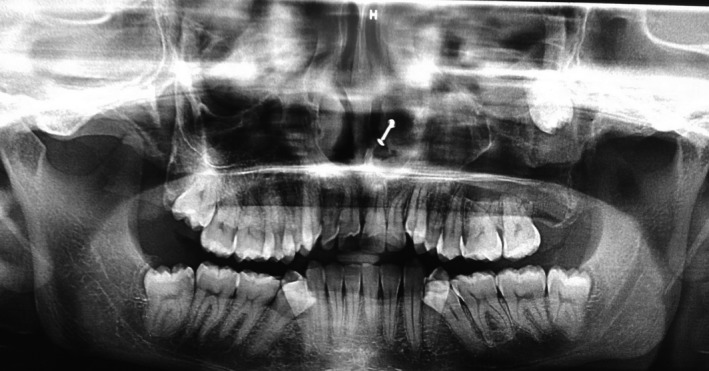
Orthopantomogram showing the presence of an ectopic tooth in the orbital floor
